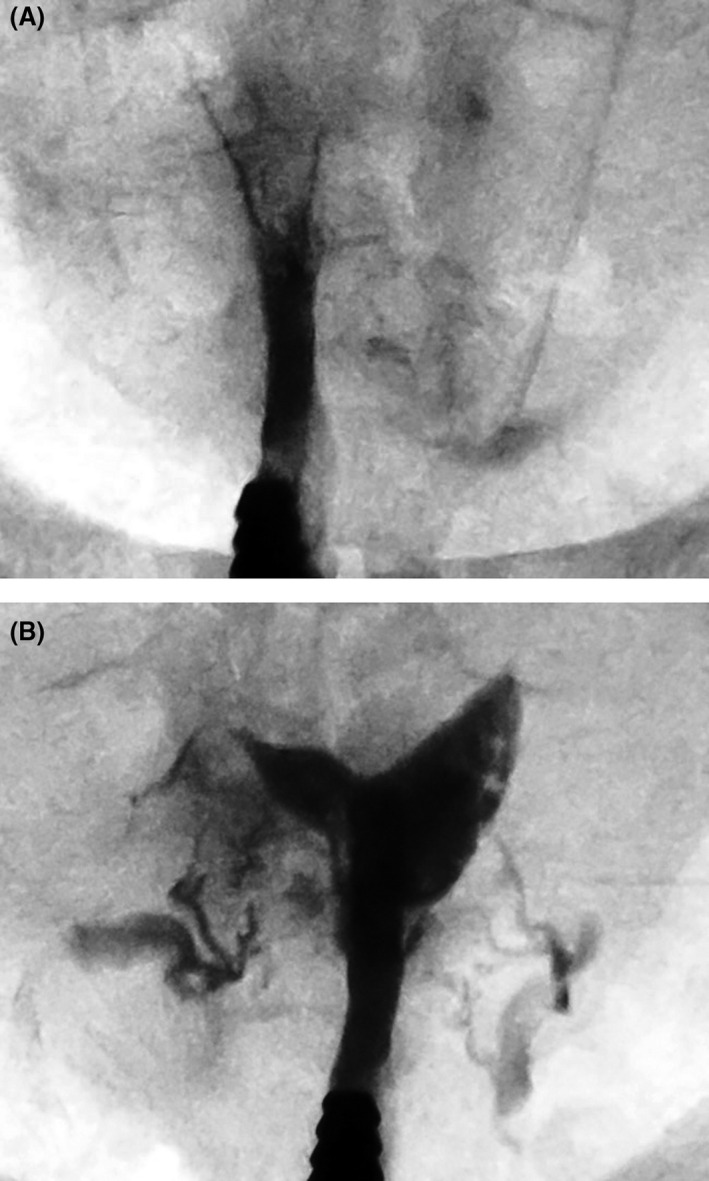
Xray images of a patient with stage III Asherman at the start (A) and the end (B) of the first of three procedures required to treat the condition.
